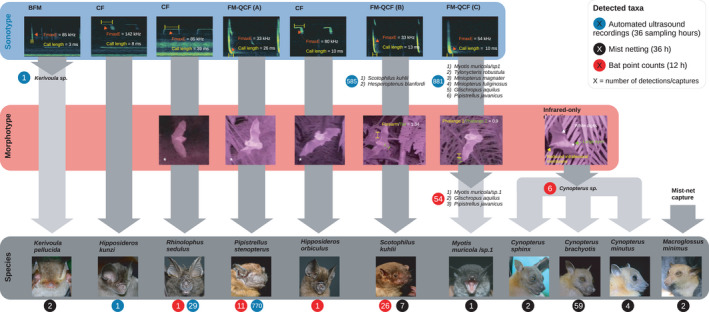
Identification workflow of bat taxa sampled in oil palm plantations in Sumatra (Indonesia) with bat point counts, as well as traditional mist‐netting and ultrasound recording methods. Call interval, start and end frequency were also used for the identification but are not shown here. Representative near‐infrared photographs are shown; they belong to sequences of multiple pictures. Asterisks denote near‐infrared imagery that was not strictly needed for identification but that was used for identity confirmation. Putative identification pathways are shown with a lighter gray tone. Photos used with permission from Ellena Yusti, Joe Chun‐Chia Huang, and Neil Jun Lobite
